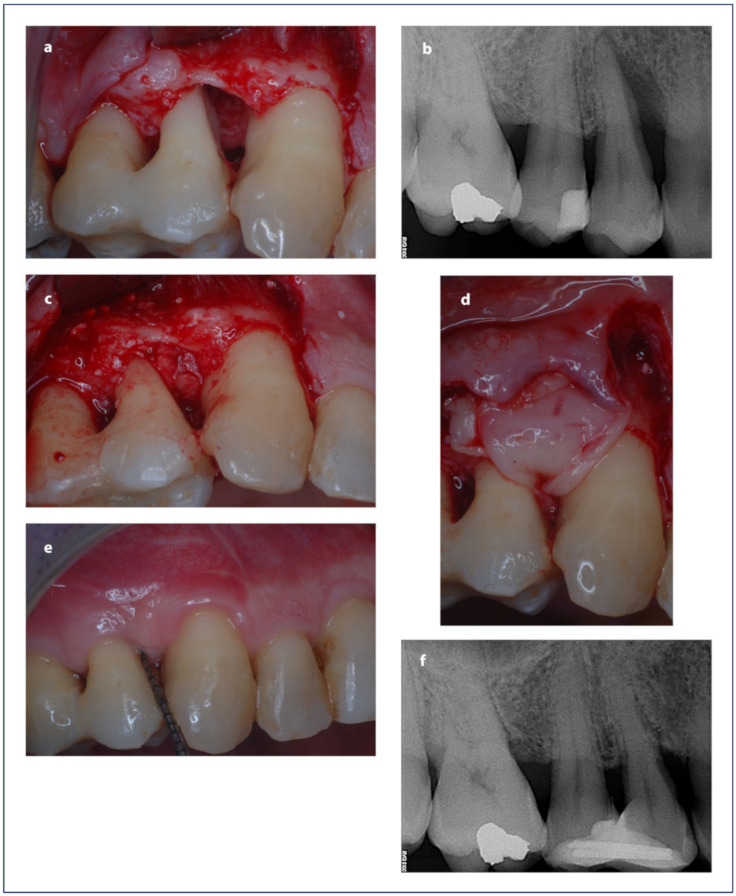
(a) The intrabony defect; (b) X-ray appearance of the defect; (c) the intrabony defect was filled with ABG mixed with L-PRF; (d) L-PRF membranes covers the graft; (e) clinical and (f) radiographic appearance of the treated site 1 year after surgery.
